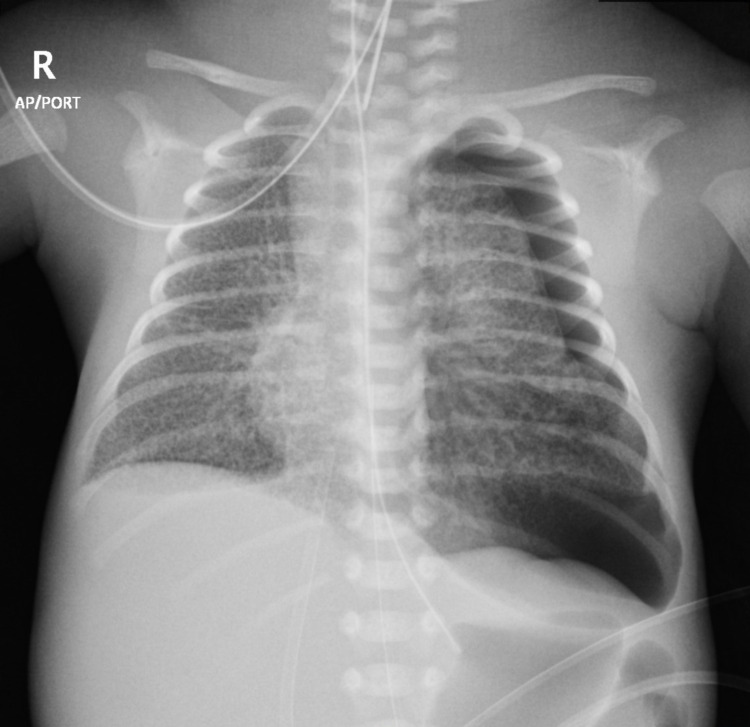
Chest radiography revealed newly developed left-sided pneumothorax.
